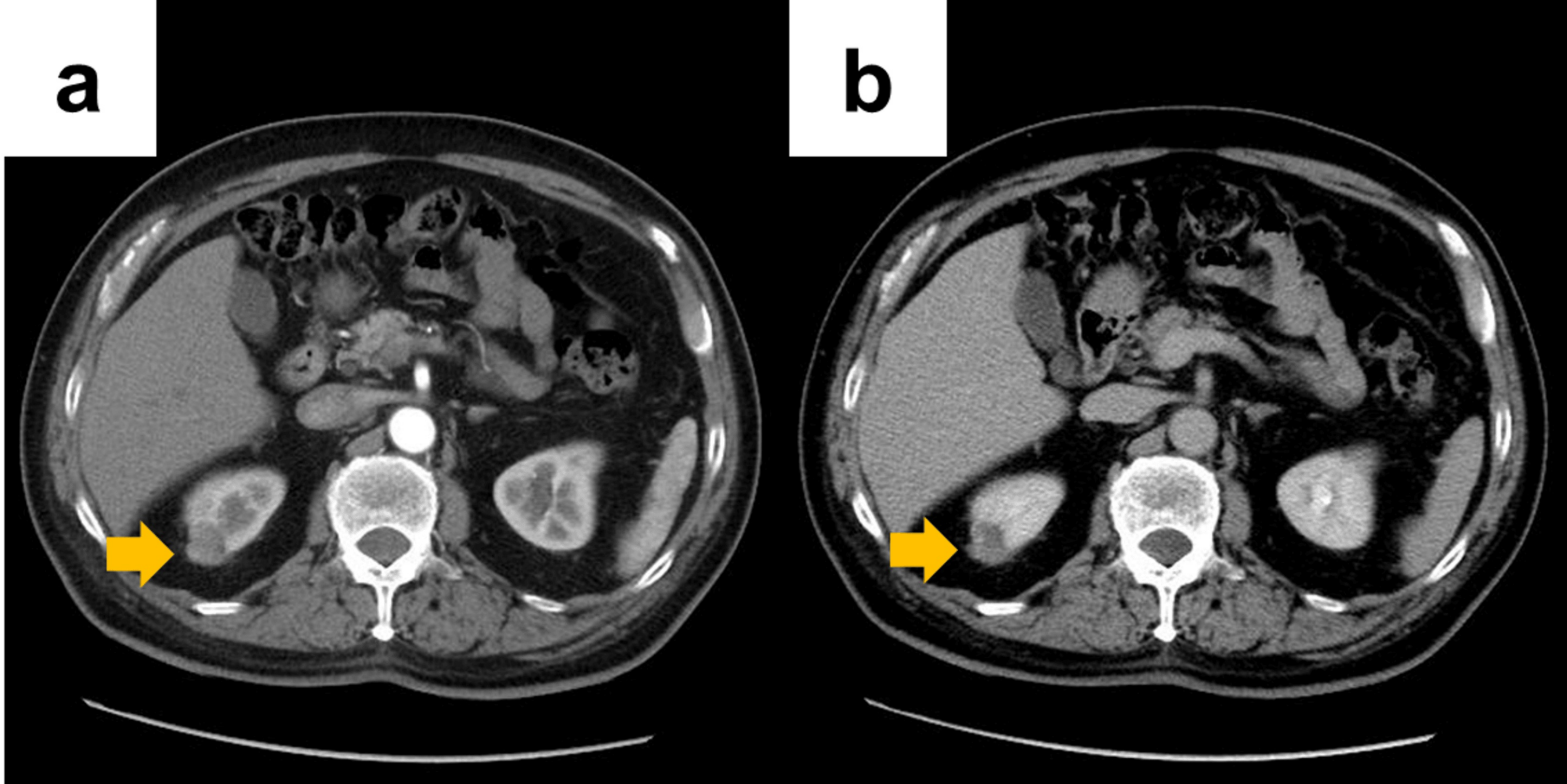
Computed tomography findings in Case 2 (a, b) Dynamic contrast-enhanced CT shows a 22-mm tumor at the lateral portion of the upper pole of the right kidney (a: early phase; b: excretory phase). Arrows indicate the renal tumor.
